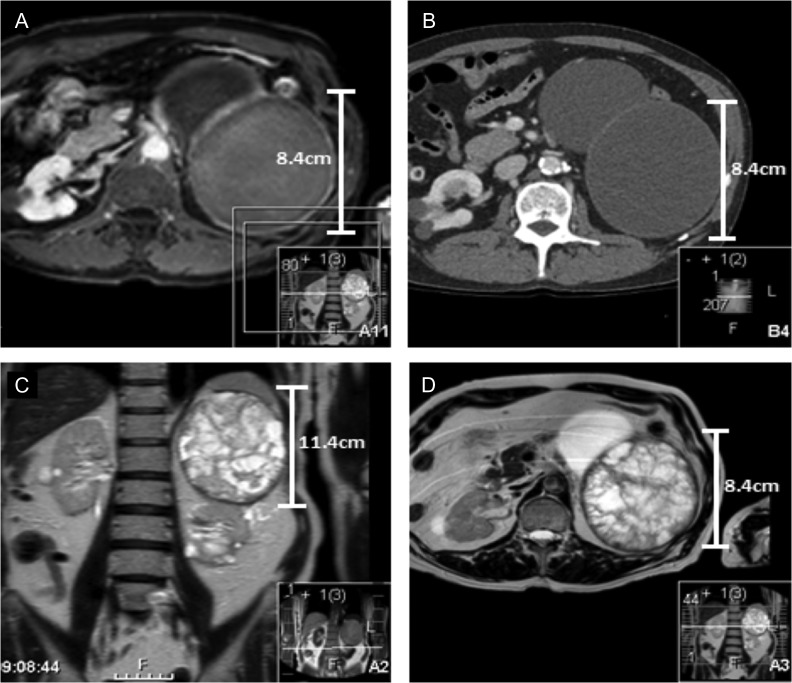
(A and B) Represent contrast and non-contrast enhanced CT scans from 2014 of the left upper pole cyst when it was noted that the patient complained of 6 months of weight loss. The CT findings indicated the upper pole cyst to still be classified as Bosniak stage IIF. There is no notable calcification, septations or convincing solid components. (C and D) Both represent the MRI of the upper pole cyst. The findings images demonstrate extensive, thick septations in the upper pole cyst upgrading the cyst to Bosniak stage III. The scans outline the limitations of surveillance CT vs MRI to stage the severity of renal cystic disease in this patient.
